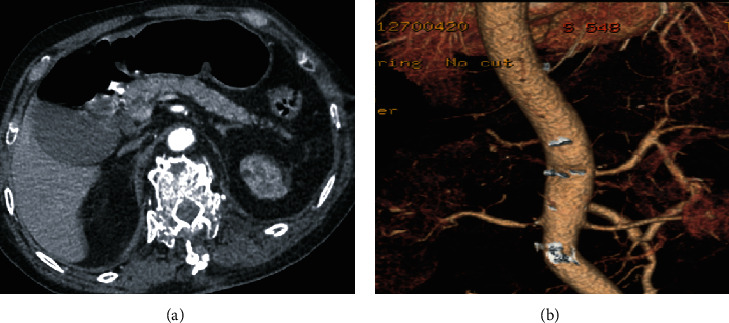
(a and b) CT angiography and three-dimensional imaging of the thoracic and abdominal aorta revealed an intramural hematoma of the abdominal aorta at the celiac trunk level.
